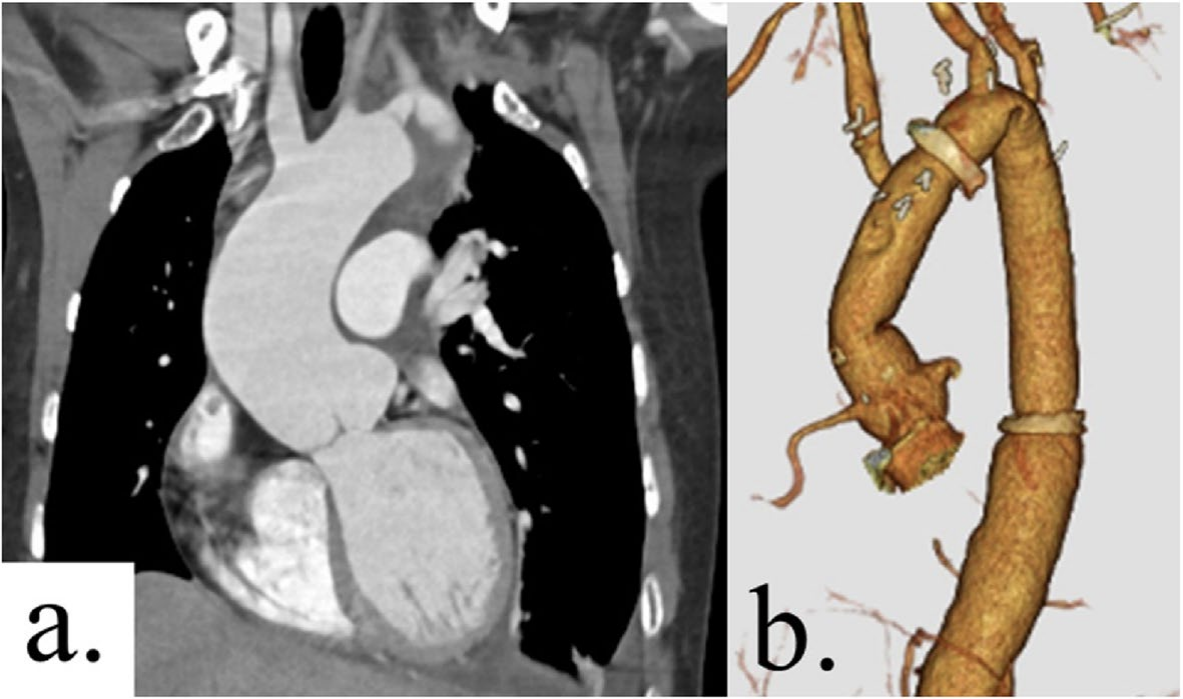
a The initial CT scan upon admission showed a dilated aortic root measuring 47 mm. b Postoperative three-dimensional CT scan
